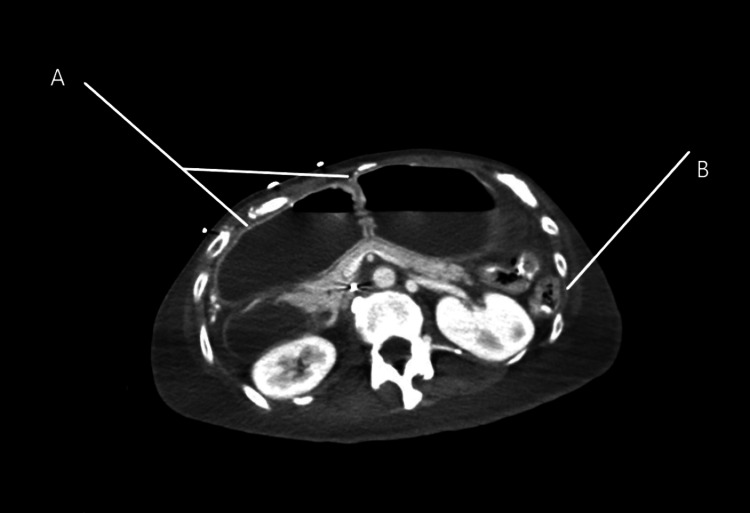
Abdominal CT, axial section, case 2. A-Dilated large bowel loops B-Decompressed descending colon
